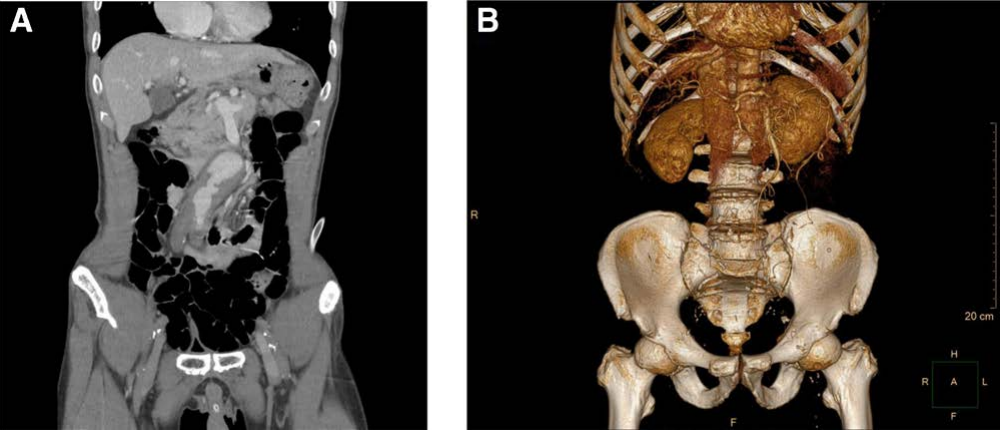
CTA showing an infrarenal AAA with thrombus formation measuring approximately 42 mm in diameter and total occlusion of the common bilateral iliac arteries. (A) Coronal image; (B) 3D reconstruction. AAA = abdominal aortic aneurysm, CTA = computed tomography angiography.
